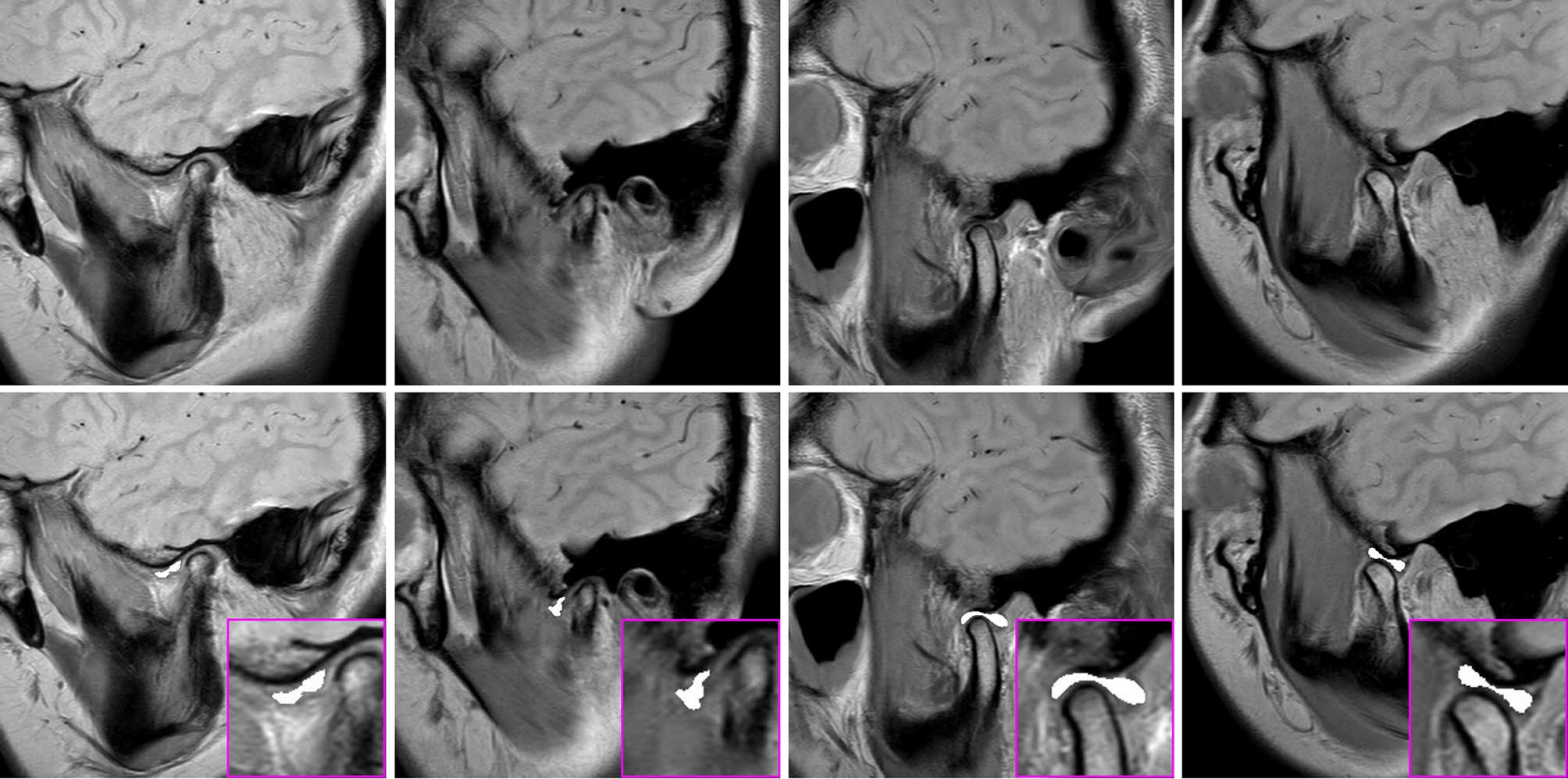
Representative images of manually segmented anterior discs. The first row shows raw MR images. The second row shows images with segmentations manually drawn by experts (white regions).
